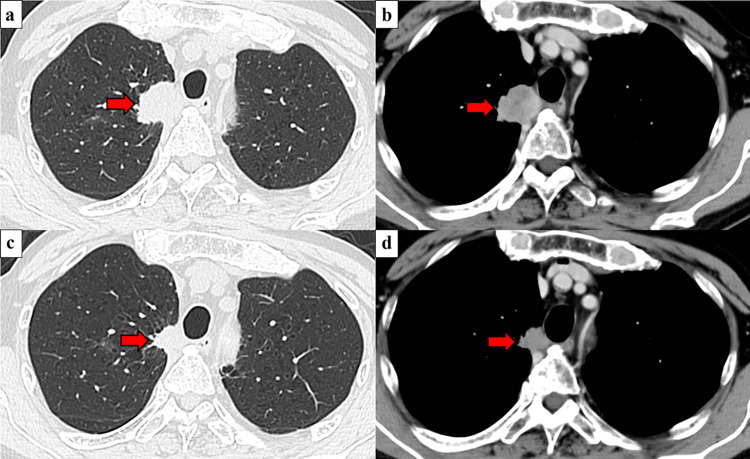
Preoperative CT findings. a. Before chemotherapy: The tumor size was 28 × 26 mm; no obvious lymph node metastases were observed. b. Before chemotherapy: Mediastinal infiltration was also observed. c. After chemotherapy: The tumor had shrunk to 18 × 17 mm. d. After chemotherapy: Mediastinal infiltration remained. The red arrow in each panel indicates the tumor.
